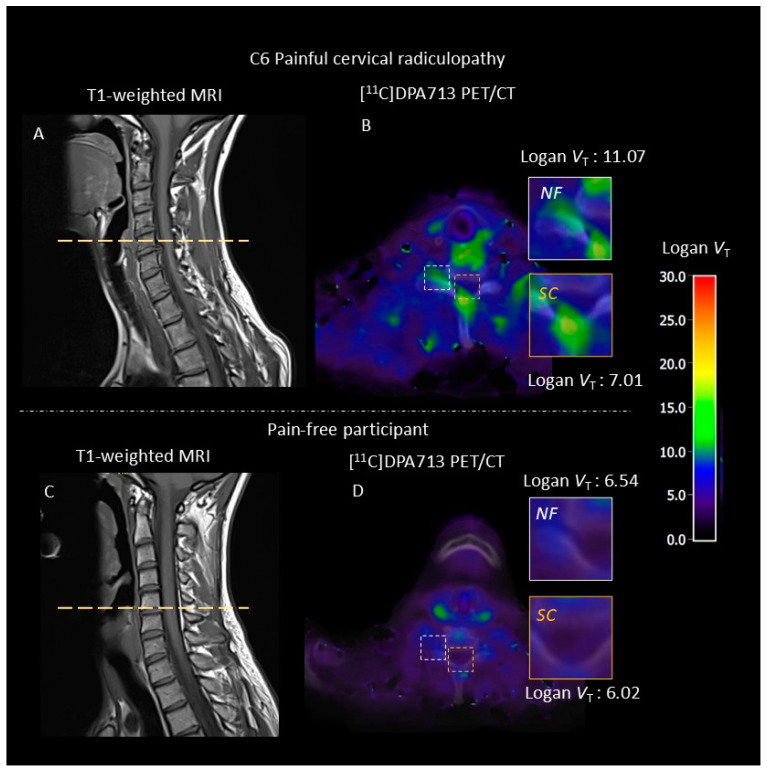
Neuroinflammation in a patient with a C6 right painful cervical radiculopathy and a pain-free participant. To quantify [^11^C]DPA713 binding at the neuroforamina and spinal cord, we utilized an image-derived input single tissue compartmental (1T2k) model. For visualization purposes, we generated volume of distribution (*V*_T_) images using Logan plot analysis, with a time threshold of t* = 30. (A): T1-weighted MRI of the patient with a painful cervical radiculopathy. The dotted line indicates the cross-sectional area used for the CT and PET analysis. (B): Parametric cross-sectional image of [^11^C]DPA713 binding merged with CT, with a zoomed view at the neuroforamina (NF) and spinal cord (SC). Higher volume of distribution (*V*_T_) indicates more tracer binding, suggesting higher levels of neuroinflammation. (C): T1-weighted MRI of a pain-free participant. (D): Follow-up parametric cross-sectional image of [^11^C]DPA713 binding merged with CT, with a zoomed view at the neuroforamina (NF) and spinal cord (SC).
